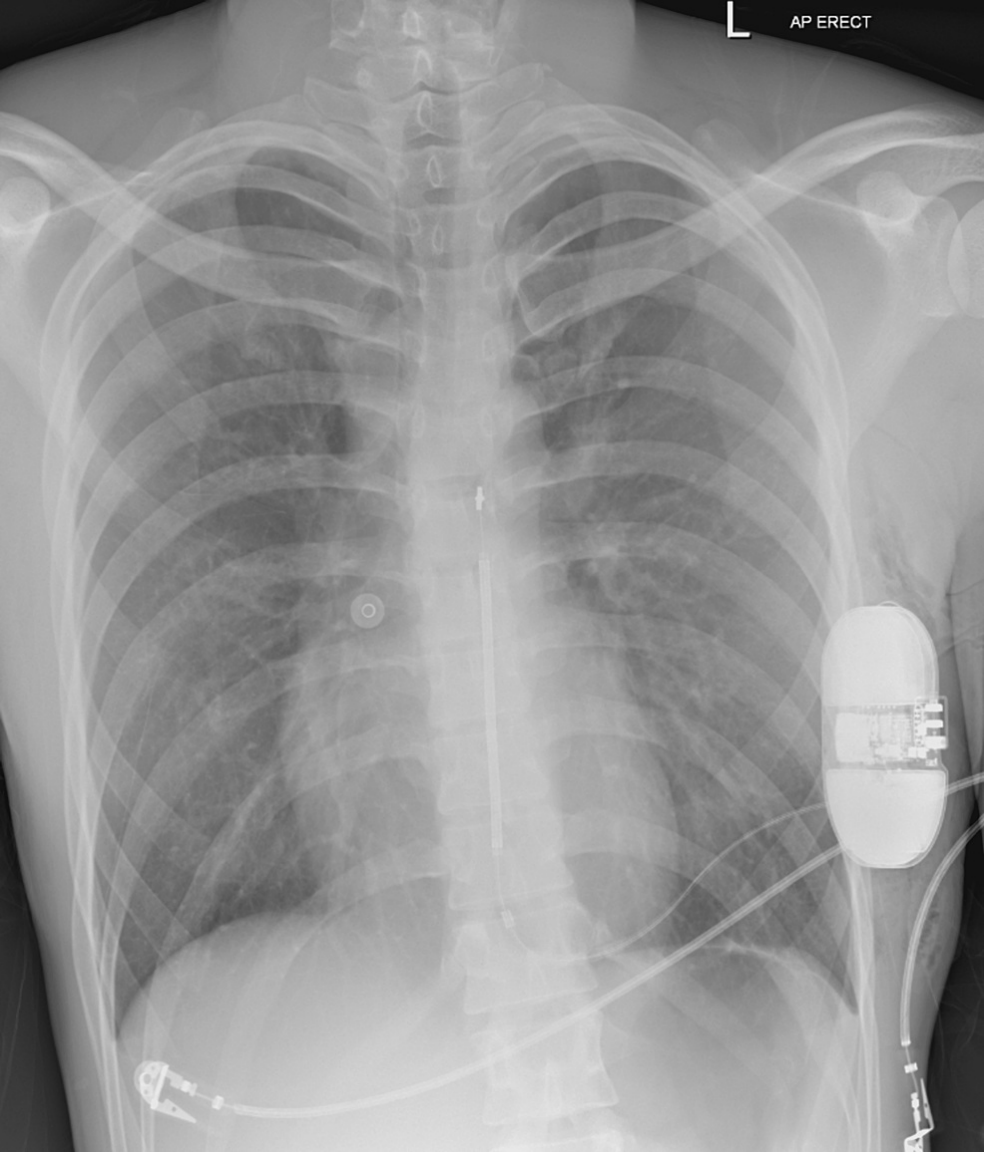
Chest radiography showing satisfactory subcutaneous implantable cardioverter defibrillator leads position
